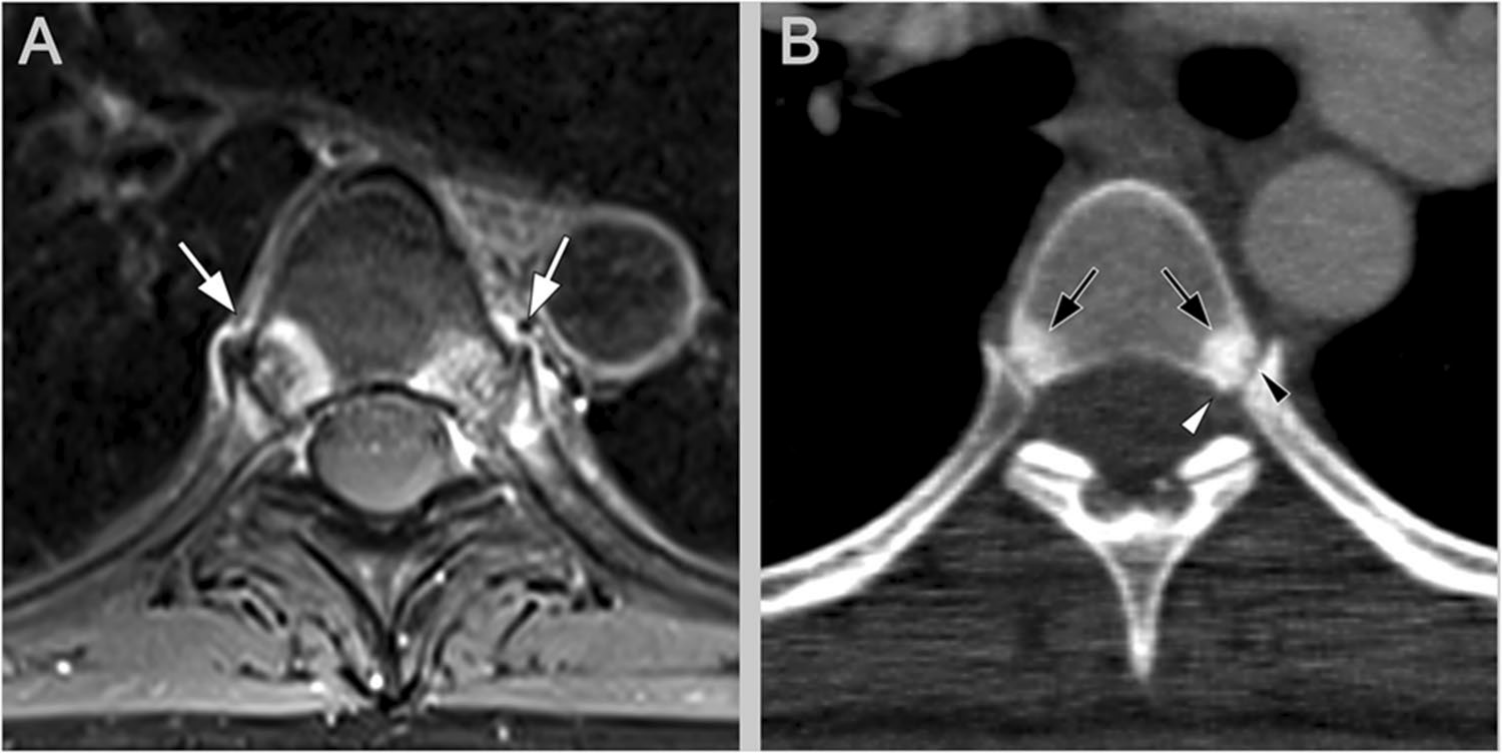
Costovertebral joint arthritis. This 37-year-old female patient with sacroiliitis and breath-dependent back pain has costovertebral joint arthritis on contrast-enhanced MRI (A, white arrows). CT (B) shows sclerosis (black arrows), erosion (black arrowhead) and periosteal proliferation (white arrowhead), typical for axSpA
